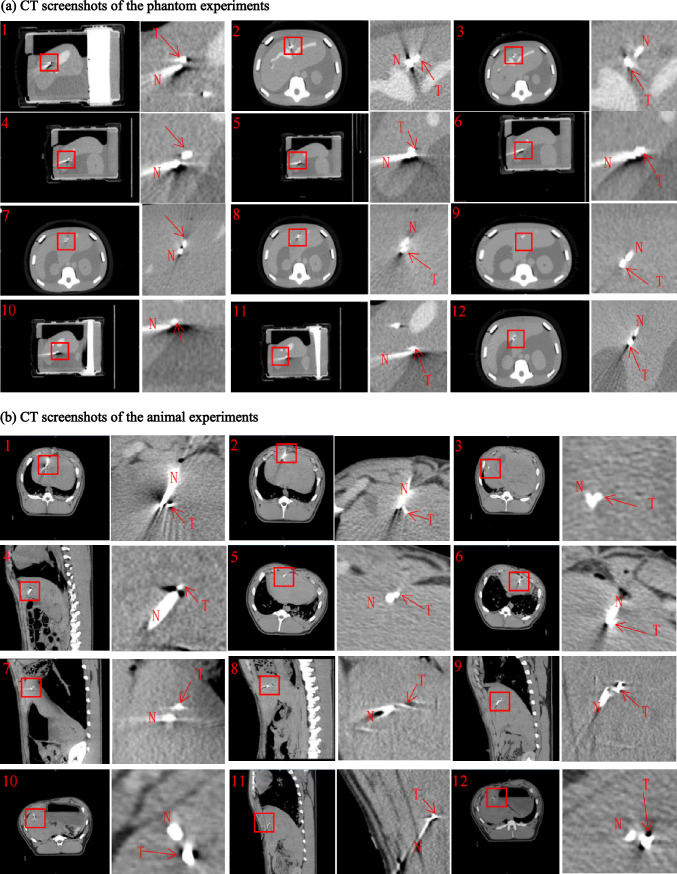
CT screenshot of the relationship between the puncture needle tip and the target point
